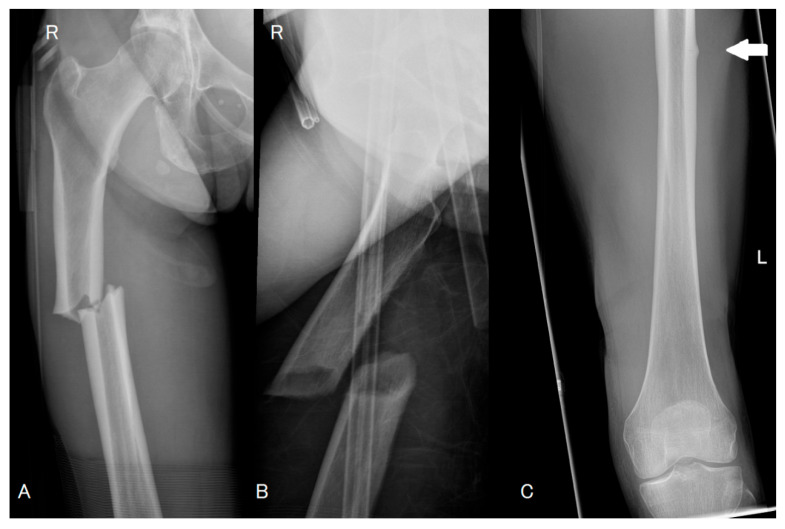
Despite these precautions, she subsequently sustained an acute injury after slipping while exiting a swimming pool and falling directly onto her right (R) side. She presented with acute right thigh pain and inability to bear weight. Plain radiographs (Panel (A,B)) demonstrated a displaced transverse fracture of the right femoral shaft with minimal comminution and characteristic lateral cortical beaking. In the context of preceding prodromal thigh pain, prior MRI evidence of bilateral stress injury, ongoing bisphosphonate therapy, and typical radiographic features, the fracture was classified as an atypical femoral fracture precipitated by low-energy trauma. She underwent surgical fixation of the right femoral fracture with intramedullary nailing [10]. Post-operatively, she progressed satisfactorily, and close surveillance of the contralateral femur was instituted in view of the well-recognised propensity for atypical femoral fractures to occur bilaterally. Following confirmation of bilateral atypical femoral fractures, ongoing bisphosphonate therapy was discontinued. Although atypical femoral fractures are most frequently associated with prolonged exposure to bisphosphonates, this case represents a recognised occurrence following a relatively shorter duration of treatment. After multidisciplinary discussion involving orthopaedic surgeons and metabolic bone specialists, anabolic therapy was initiated with teriparatide (parathyroid hormone 1–34) at a dose of 20 micrograms daily, planned for a total duration of two years, in conjunction with continued calcium and vitamin D supplementation [11,12]. Given the presence of an incomplete atypical fracture (white arrow, Panel (C)) in the contralateral femur (Left (L) side) and the persistent risk of progression to a complete fracture, prophylactic intramedullary nailing of the left femur was recommended and is planned in the near future [11,13]. Denosumab represents an alternative antiresorptive option in postmenopausal osteoporosis, supported by high-level evidence (Level I) for reduction in vertebral, non-vertebral, and hip fractures, and available clinical trial data indicate that it does not adversely affect fracture healing [14,15]. The long-term management strategy includes repeat dual-energy X-ray absorptiometry following completion of anabolic therapy and careful reassessment of future osteoporosis treatment, with the aim of optimising fracture prevention while minimising the risk of further atypical femoral injury. After completion of teriparatide, sequential therapy with an antiresorptive agent is planned to consolidate BMD gains and maintain anti-fracture efficacy; in the present case, depending on DXA response and residual fracture risk, transition to denosumab will be considered.
